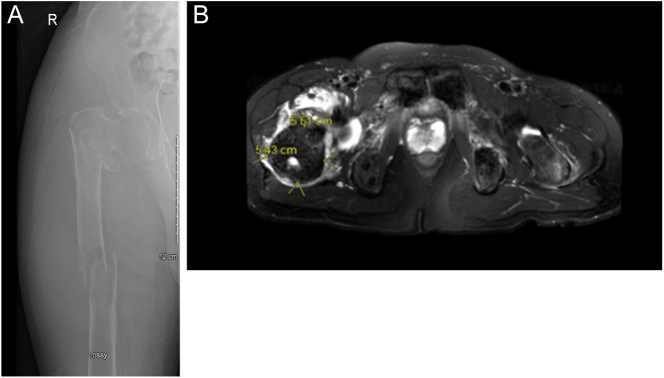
(A) Femoral X-ray: right proximal femoral diaphyseal pathologic obliquely
